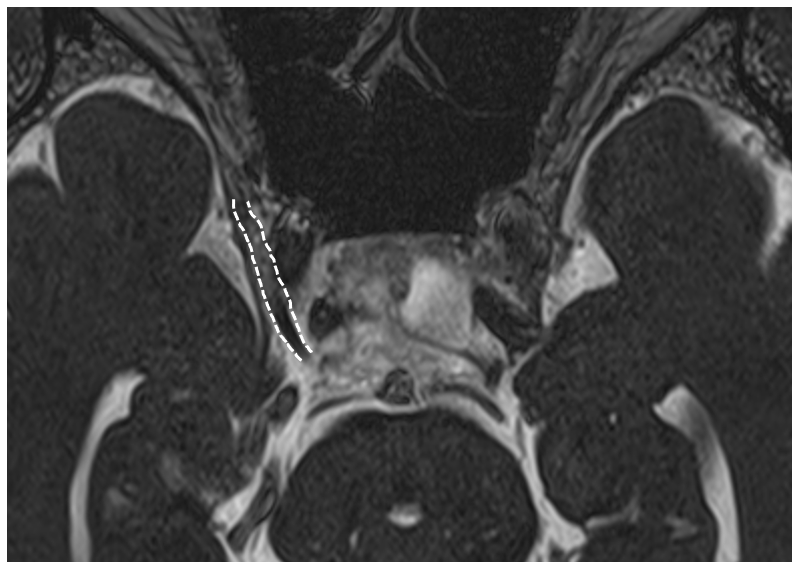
Contrast-enhanced CISS-MRI demonstrates the swelling and enhancement in the right oculomotor nerve (indicated by
